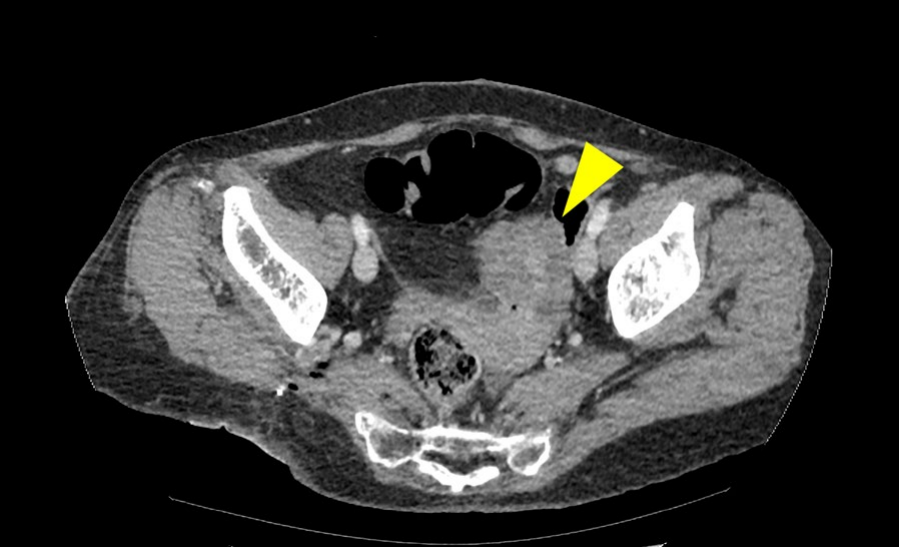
A contrast-enhanced CT scan showing a low-density lobulated tumor in the pelvis adjacent to the small intestine
